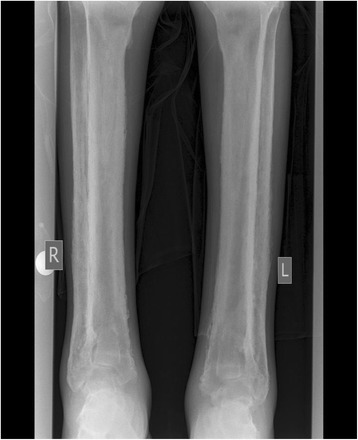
X-rays of tibia and fibula showing periosteal thickening
